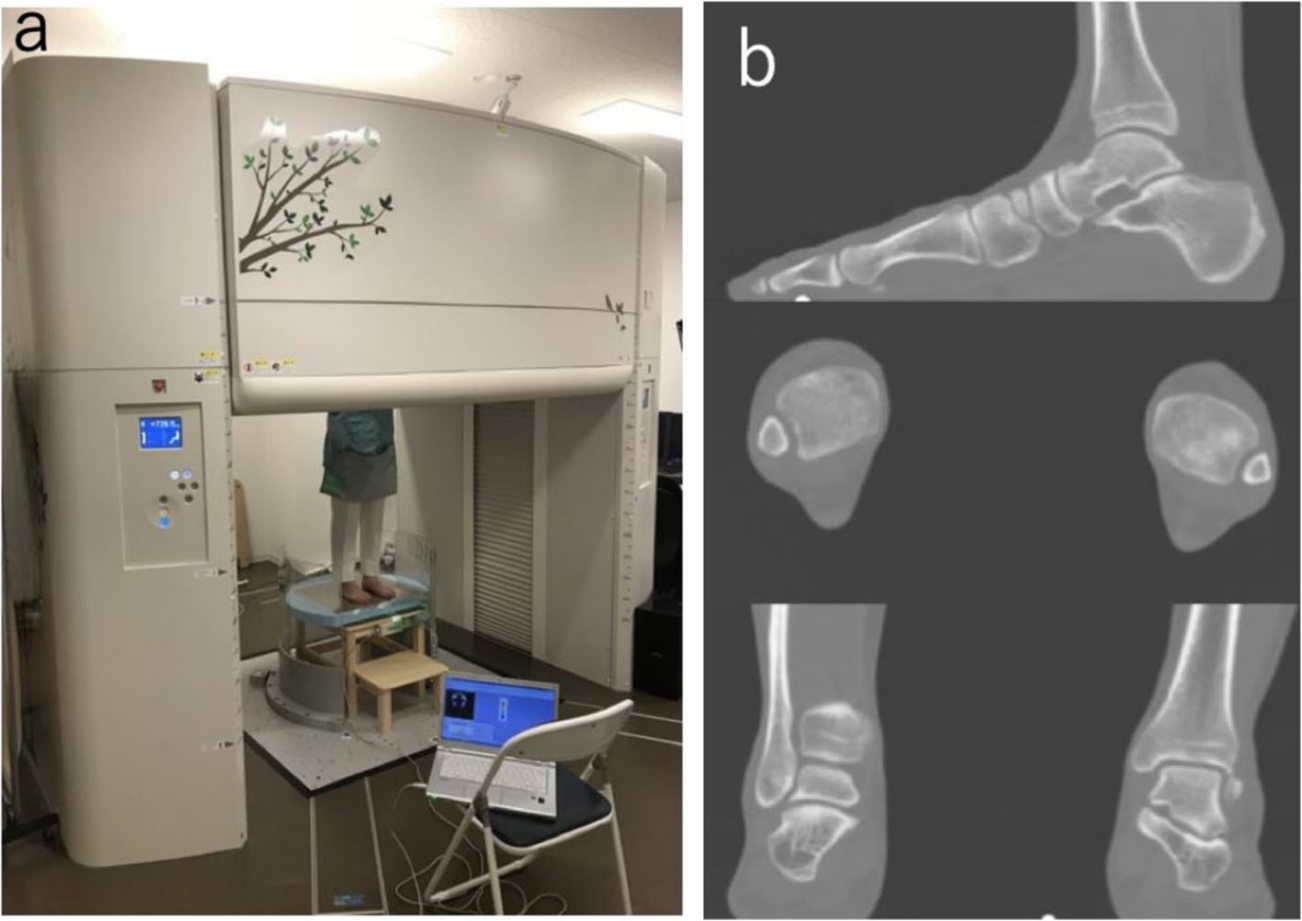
320-row upright computed tomography (CT) scanner. a The CT images were acquired from the distal femur to the entire foot using a 320-row upright CT scanner (prototype TSX-401R; Canon Medical Systems, Otawara, Japan). The condition of each weightbearing stance was measured using a pressure mat (BIG-MAT; NITTA Corporation, Osaka, Japan) and pressure calculation system (FootMat; Tekscan, South Boston, MA, USA). b The image qualities of the upright CT scanner are good to excellent
